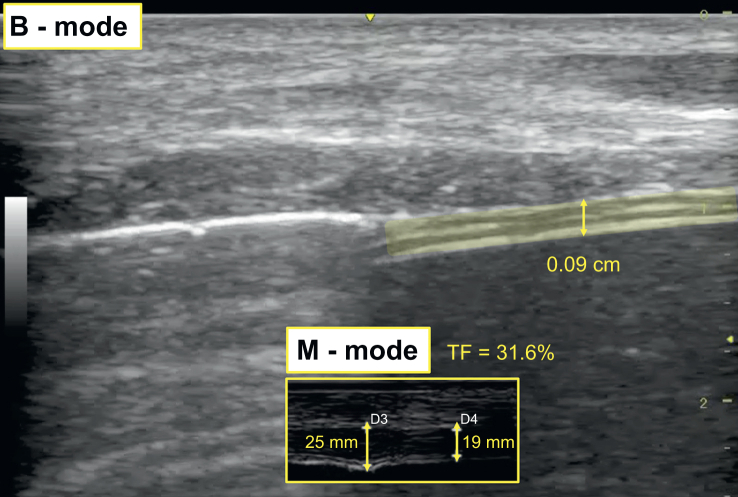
Diaphragmatic thickening. Ultrasonographic evaluation revealed diaphragmatic atrophy, observing a decrease in diaphragm thickness during expiration (0.09 cm; normal value: 0.13–0.15 cm), and a thickening fraction of 31.6%. Diaphragmatic thickening is seen with a 7.5–10 MHz linear probe at the level of the apposition zone. The end-expiratory thickness in expiration was 19 mm, whereas the end-inspiratory thickness in inspiration was 25 mm. The thickening fraction corresponded to: TF=(25–19)/19×100=31.6%.
